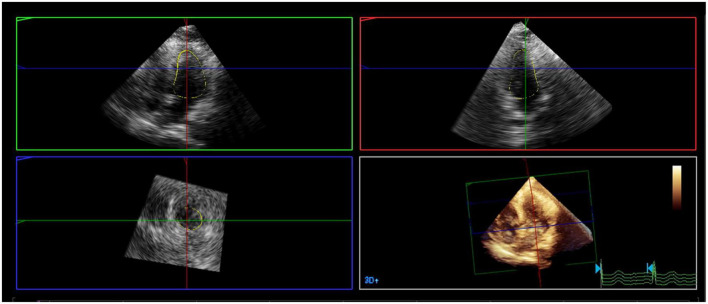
A mathematical full-volume model of the left ventricle by real-time three-dimensional echocardiography.
